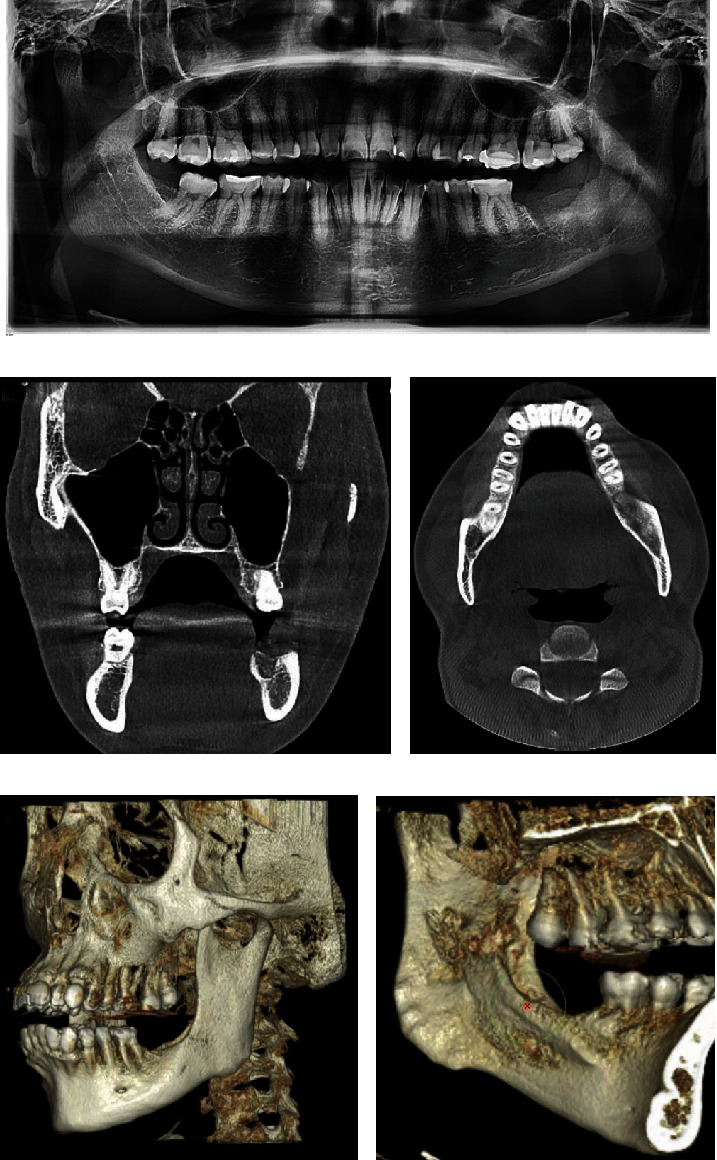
Posttreatment imaging studies. (a) 7-day postoperative panoramic radiograph demonstrating the socket of the second molar. (b, c) 4-month postoperative CT scan and coronal and axial cuts revealing increased radiodensity at the surgical site. (d, e) Isovolumetric reconstruction showing the integrity of the buccal and lingual cortical bone.
